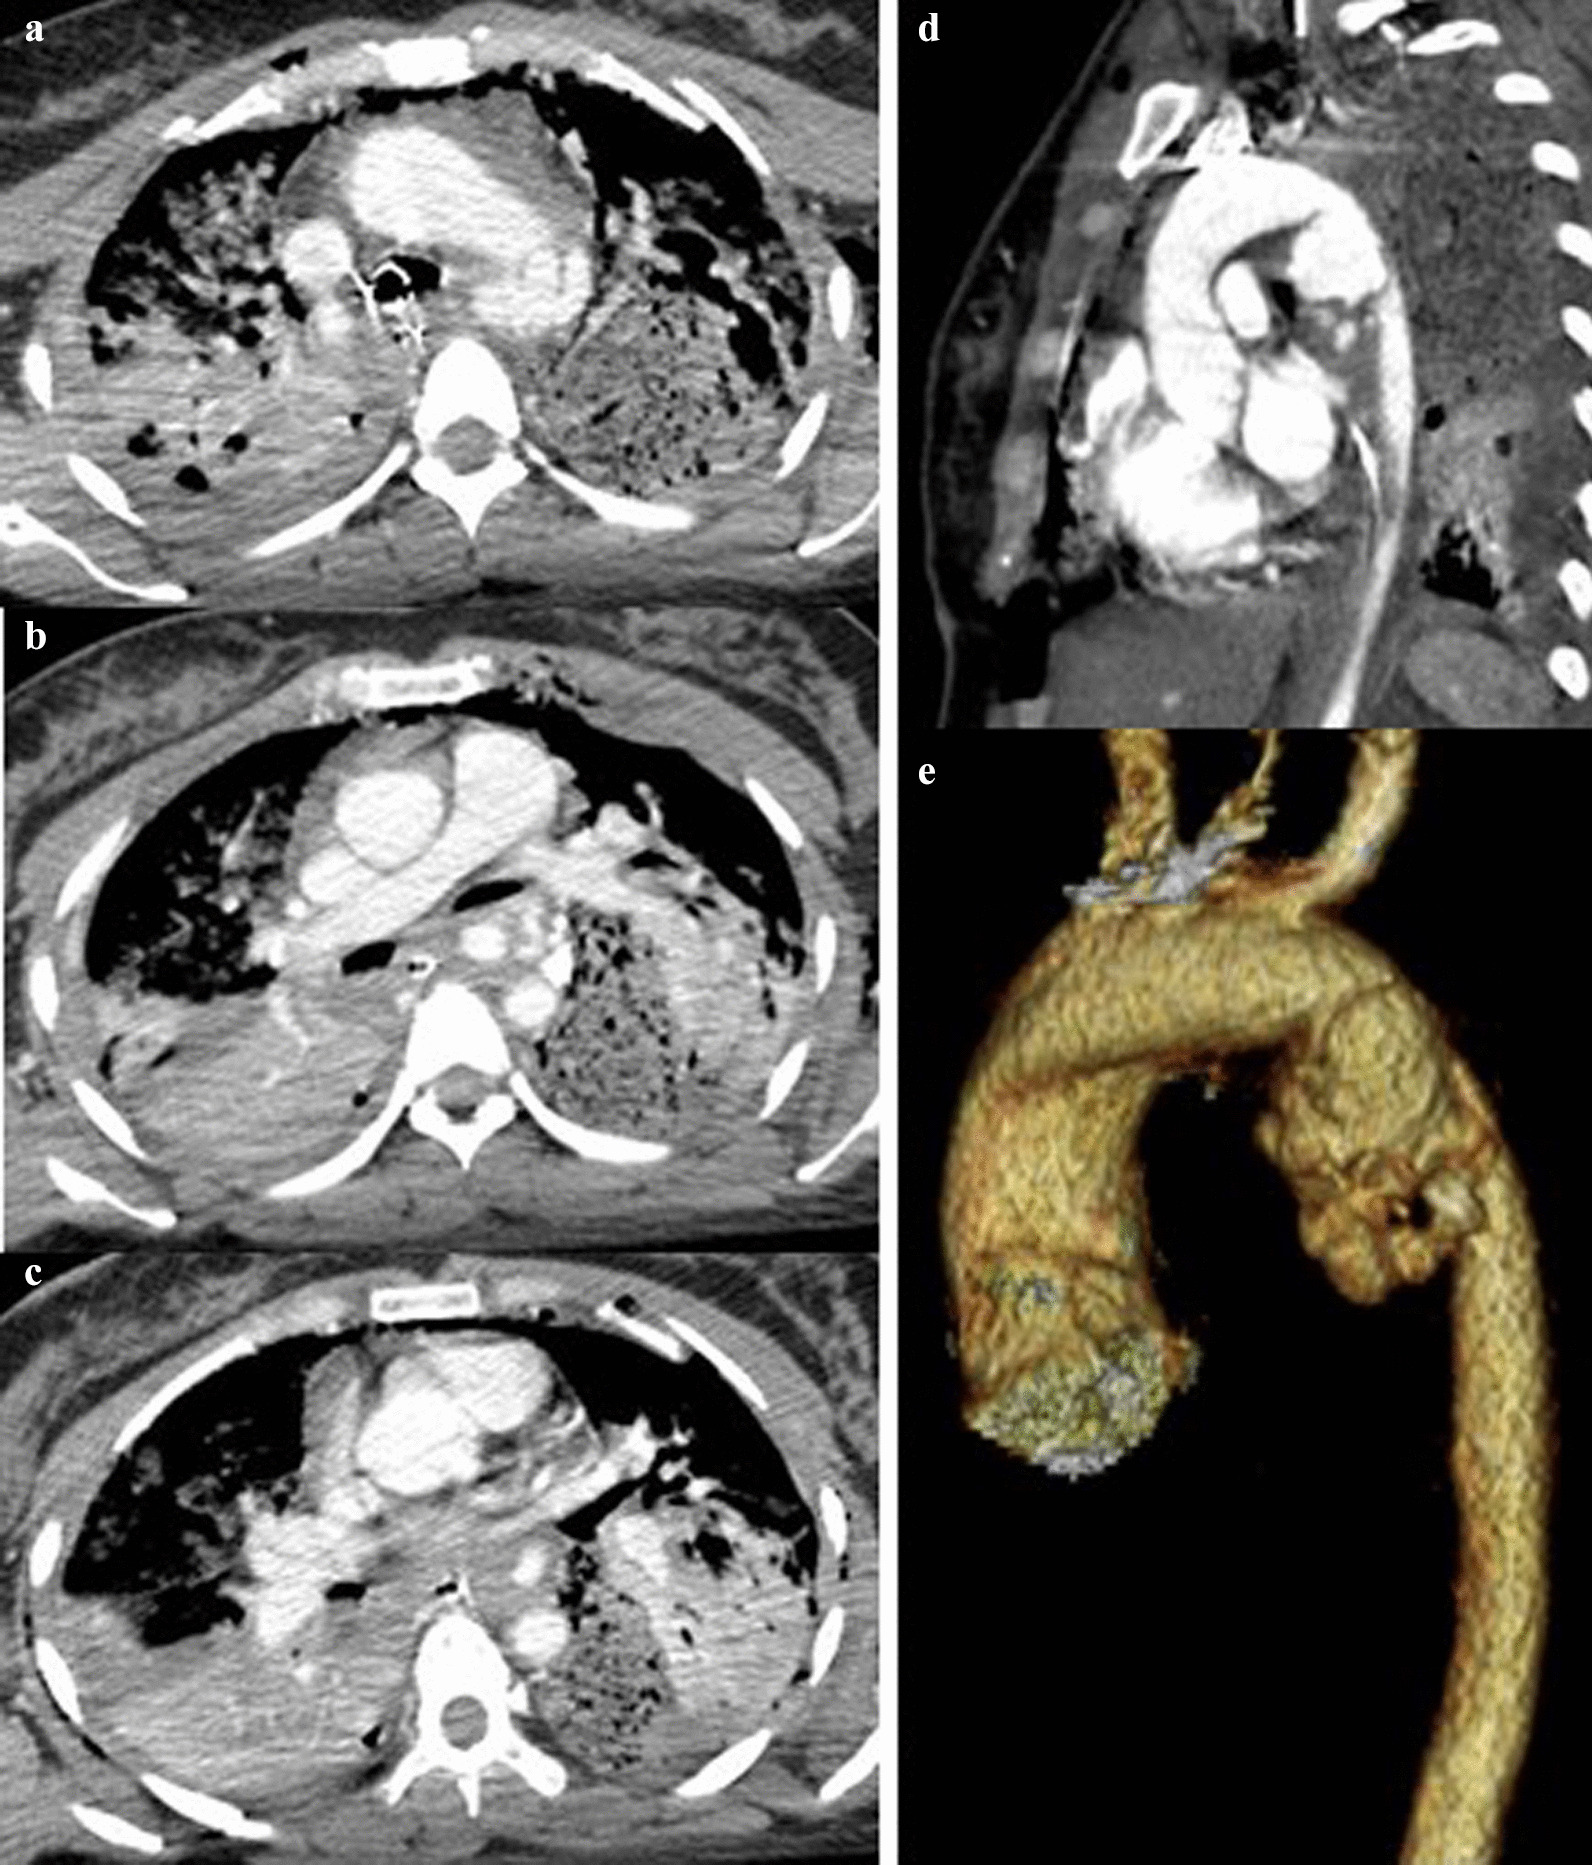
Preoperative contrast enhanced computed tomography of thoracic aortic injury. a–c Axial views, d Sagital view, e 3D Multiplanar reconstruction of aorta. Contrast enhanced computed tomography shows the aortic injury of isthmus and extravasation of contrast media into the mediastinum. Massive hemothorax was also observed in the bilateral thoracic cavity
